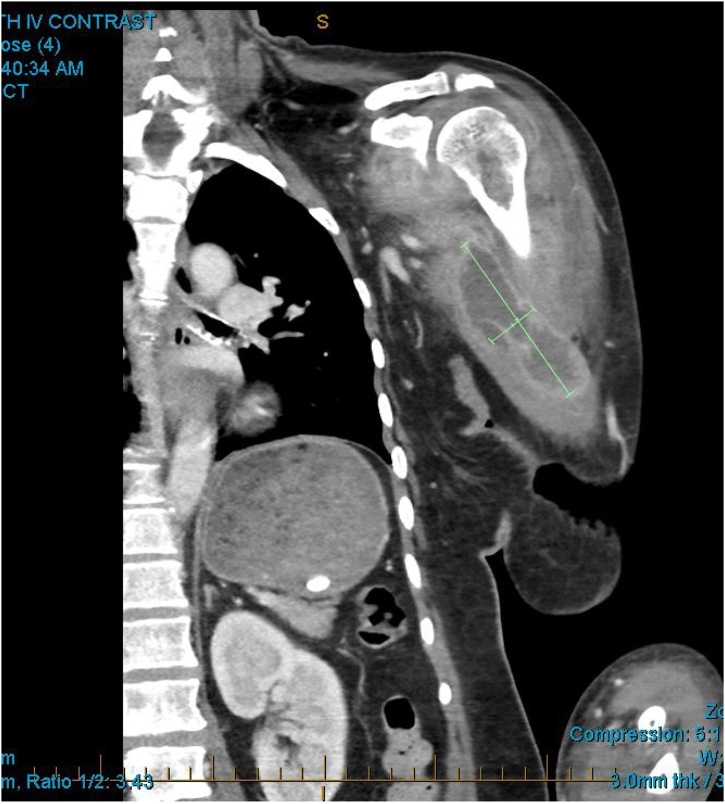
Computed tomography showed a large intramuscular abscess in the biceps brachii.
